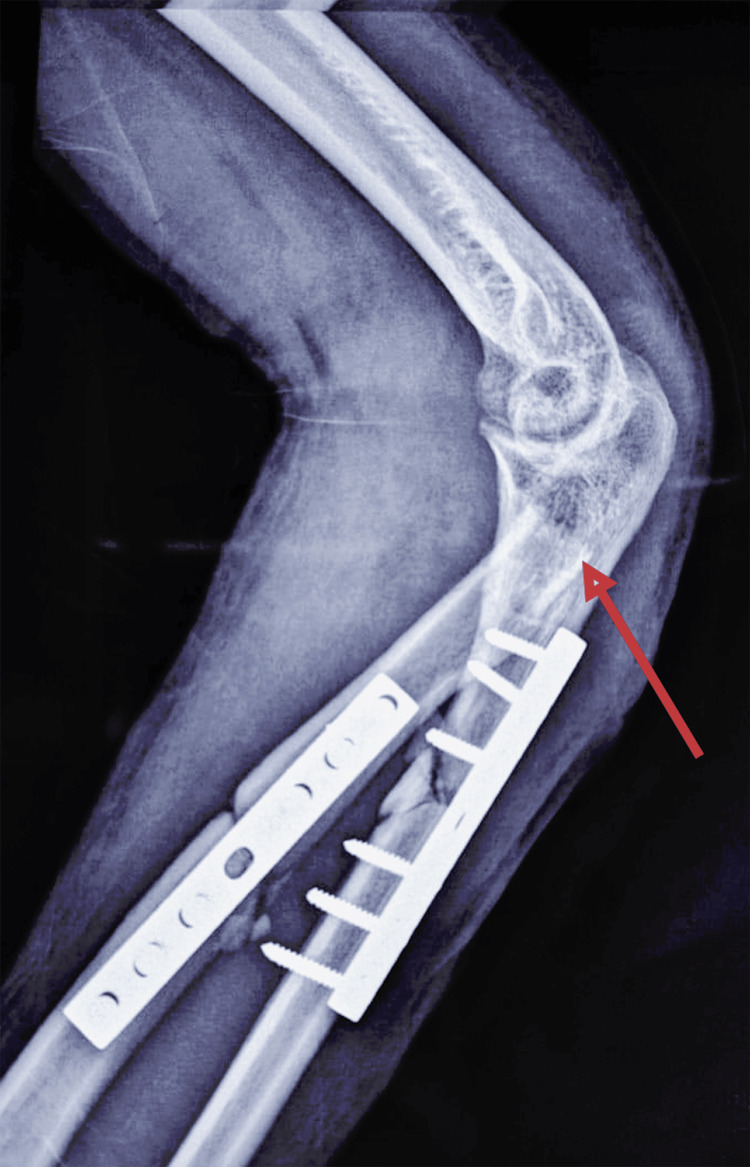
Postoperative lateral radiograph of the left elbow showing radial head resection
